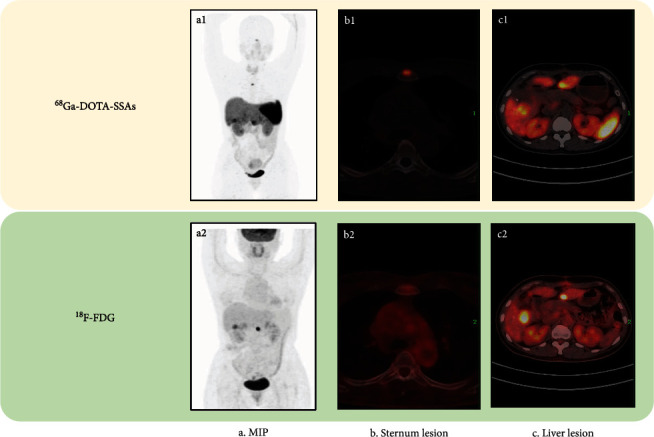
Dual-tracer imaging in a 46-year-old woman with NET G3 (Ki-67 index 22%) accompanied by liver, lymph node, and bone metastases of unknown primary origin: (a1, a2) Maximum intensity projection of dual-tracer imaging; (b1, b2) Sternum lesion with somatostatin receptor (SSTR) expression on ^68^Ga-DOTA-SSAs PET/CT but no bone metastases were detected by ^18^F-FDG PET/CT; (c1, c2) ^18^F-FDG PET/CT imaging of liver lesions showing liver metastases (LMs) with higher FDG metabolism, while ^68^Ga-DOTA-SSAs PET/CT imaging showed LMs with lower SSTR expression.
